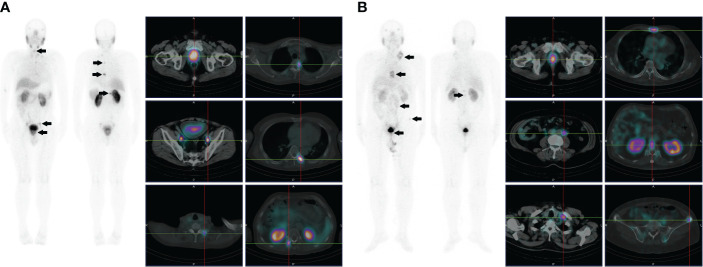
(A) Images of a 72-year-old male with high-risk prostate cancer (PSA, 188.7 ng/ml; ISUP grade 5). Whole-body planar scintigraphy (indicated by the black arrow) and transaxial SPECT/CT fusion images showed abnormally increased PSMA uptake in prostate cancer (SUVmax 22.5) and multifocal PSMA avid distant metastatic disease (including pelvic lymph node and bone metastases). (B) Images of a 68-year-old male with high-risk prostate cancer (PSA, 90.3 ng/ml; ISUP grade 5). Whole-body planar scintigraphy (indicated by the black arrow) and transaxial SPECT/CT fusion images revealed abnormally increased PSMA uptake in prostate cancer (SUVmax 13.1) and multifocal PSMA-avid distant metastatic disease (including pelvic and extrapelvic lymph nodes and bone metastases).
